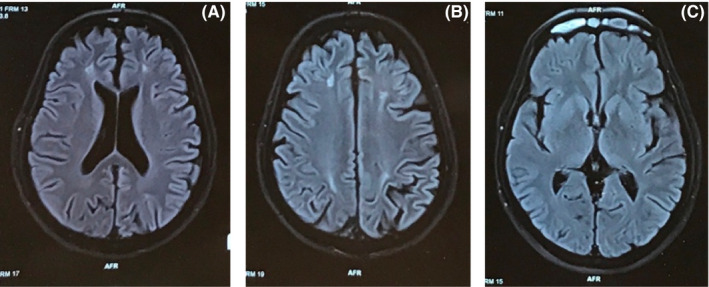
(A) Axial fluid‐attenuated inversion recovery (FLAIR) MRI brain image shows ill‐defined hyperintense areas in deep white matter of bilateral frontal lobes. (B) Axial fluid‐attenuated inversion recovery (FLAIR) MRI brain image shows ill‐defined hyperintense areas in deep white matter of bilateral frontal and parietal lobes. (C) Axial fluid‐attenuated inversion recovery (FLAIR) MRI brain image shows normal bilateral basal ganglia, thalamus, and cortical gray matter
